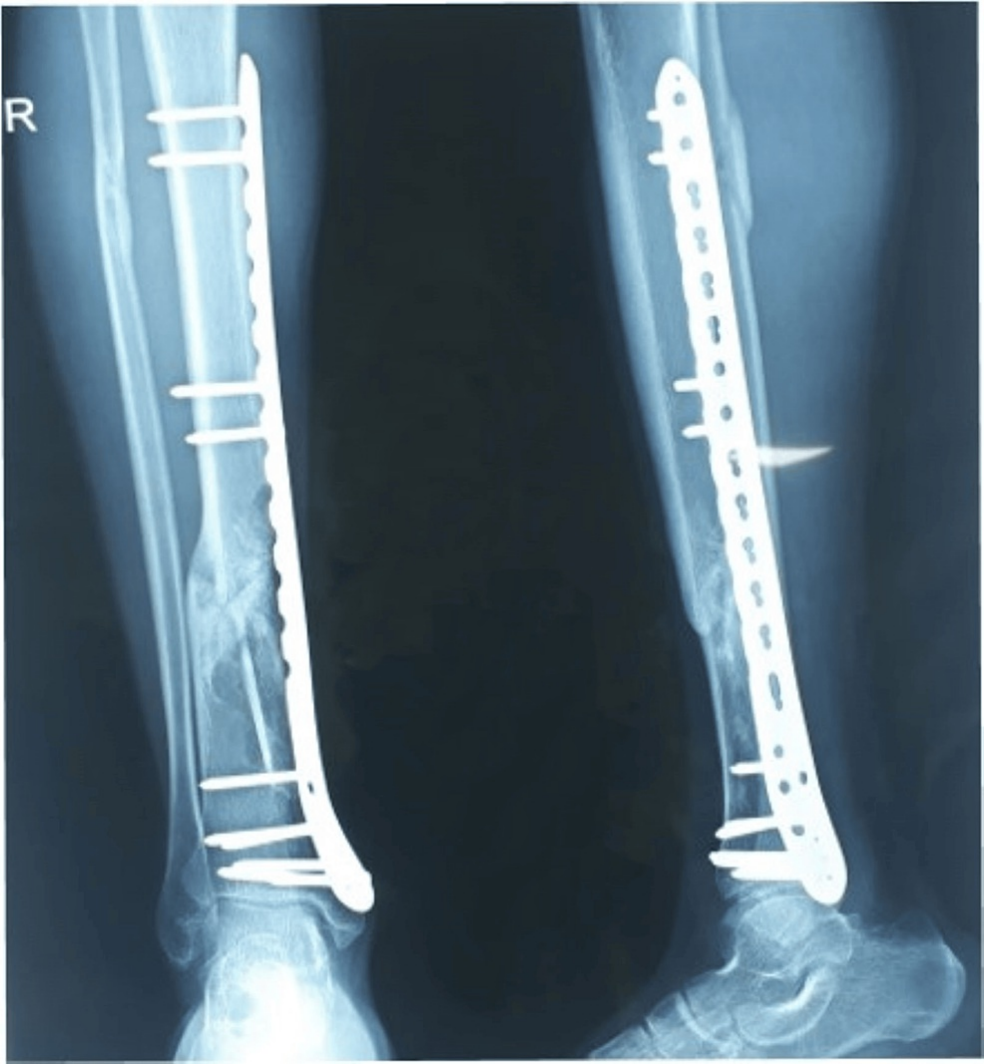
Follow up radiograph at one year showing complete union
